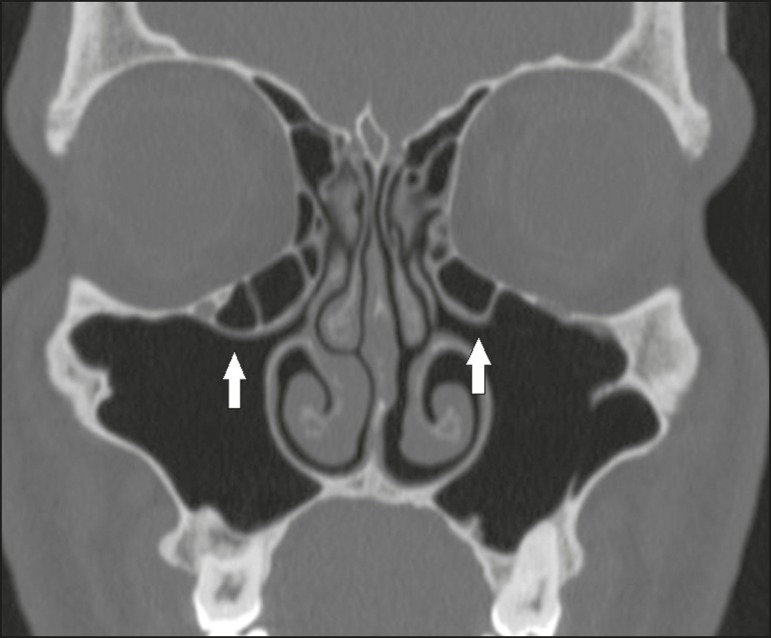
Coronal CT slice showing Haller cells, bilaterally (arrows), that are
